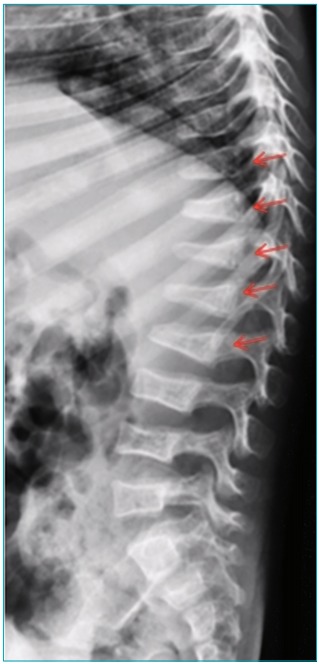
Female patient with osteogenesis imperfecta type 4. Radiographic image of the thoracolumbar spine showing multiple vertebral fractures.
